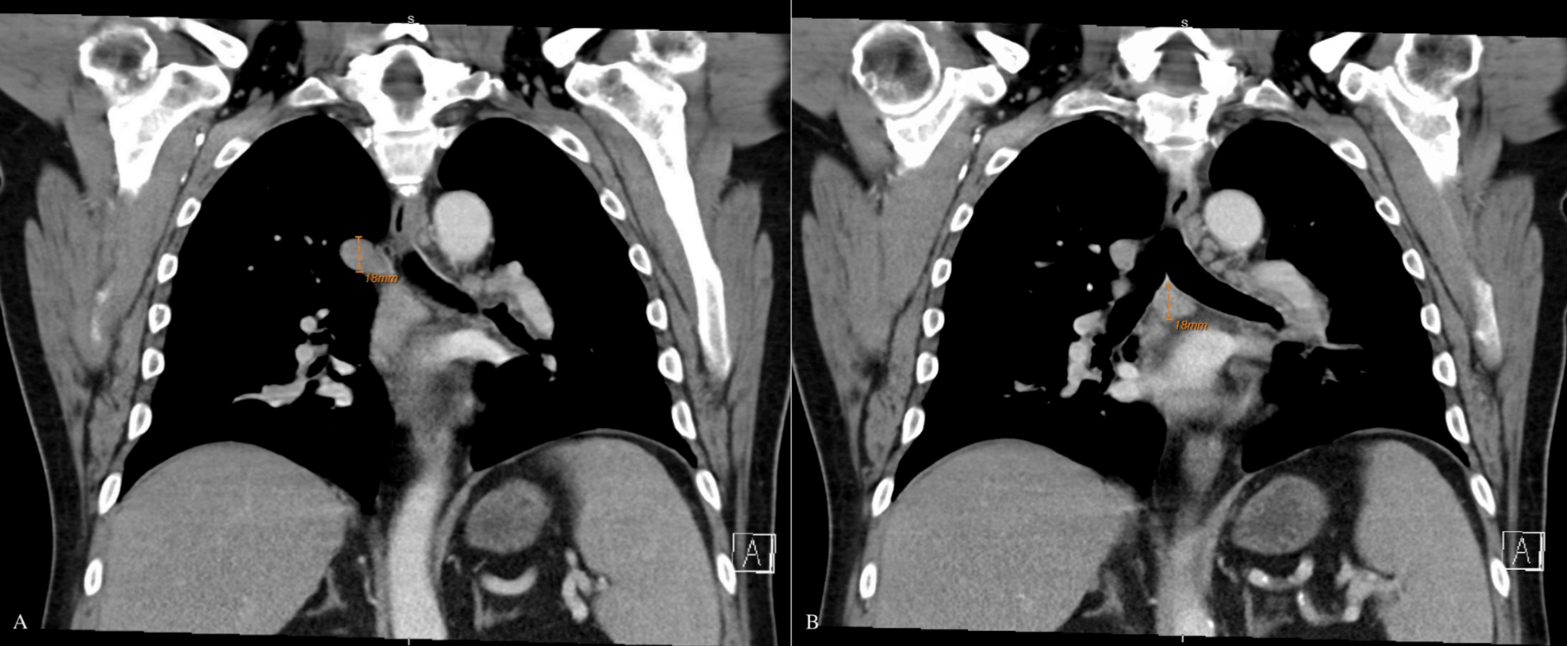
CT chest. A) CT chest showing hilar lymph node B) CT chest showing pre-carinal lymph node. CT: Computed tomography.
